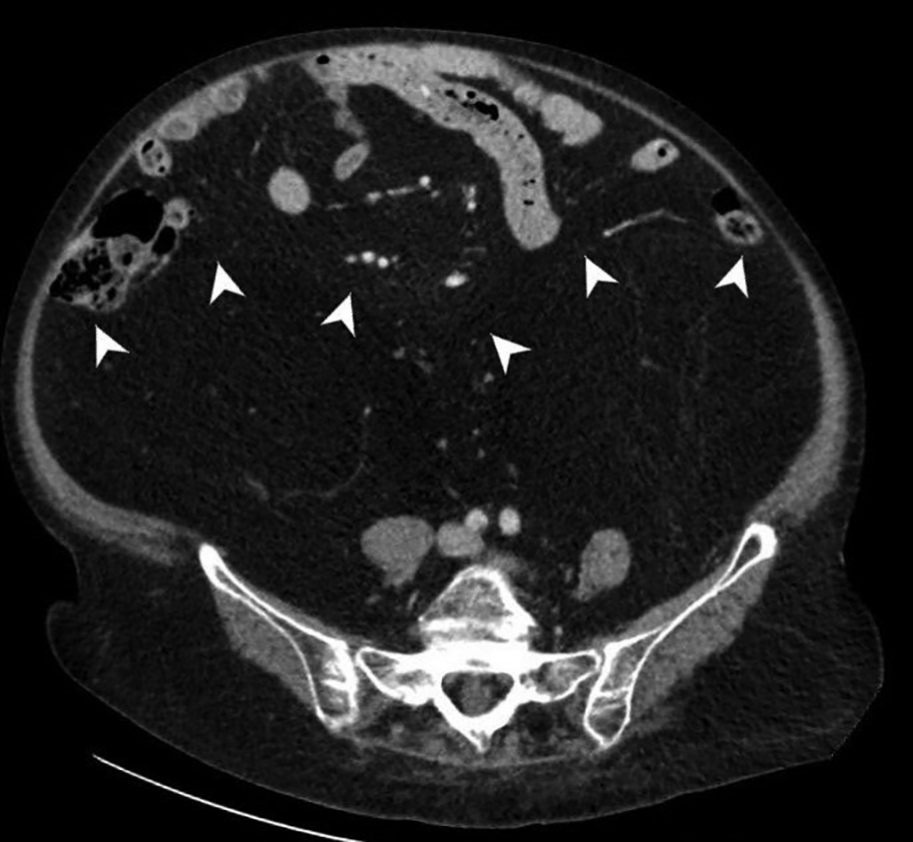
CT shows symmetrical and homogeneous increased retroperitoneal fat.
